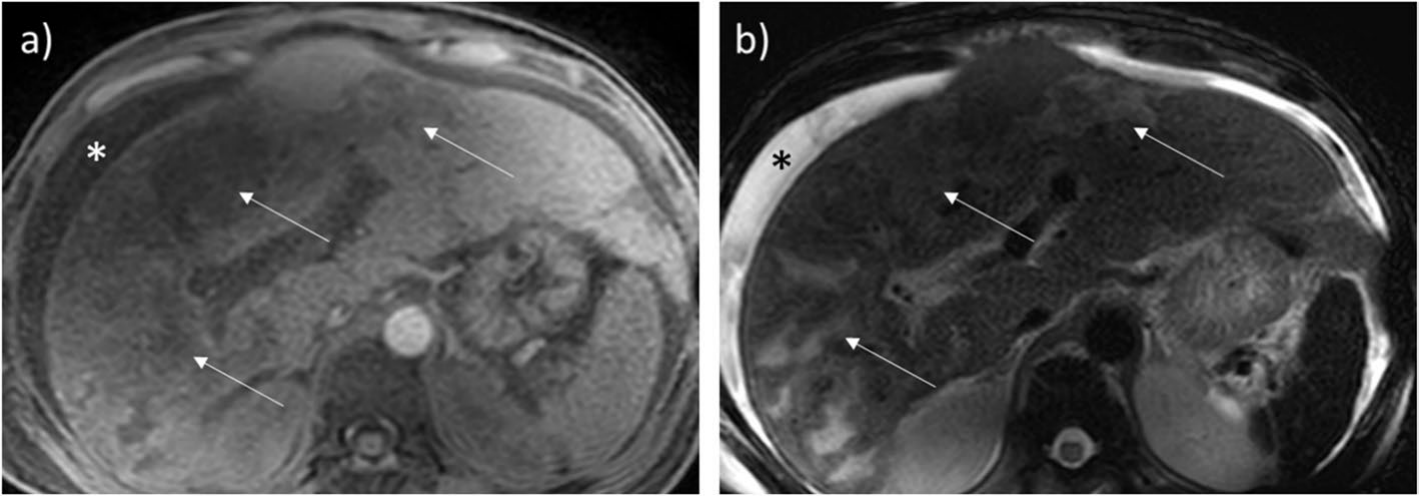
MRI evidence of necrosis due to HELLP in a 32-year-old patient. Necrosis appears as multiple slightly hypointense bands on T1w sequences (a), corresponding to areas of T2 hyperintensity (b). Ascites is also seen (*)
